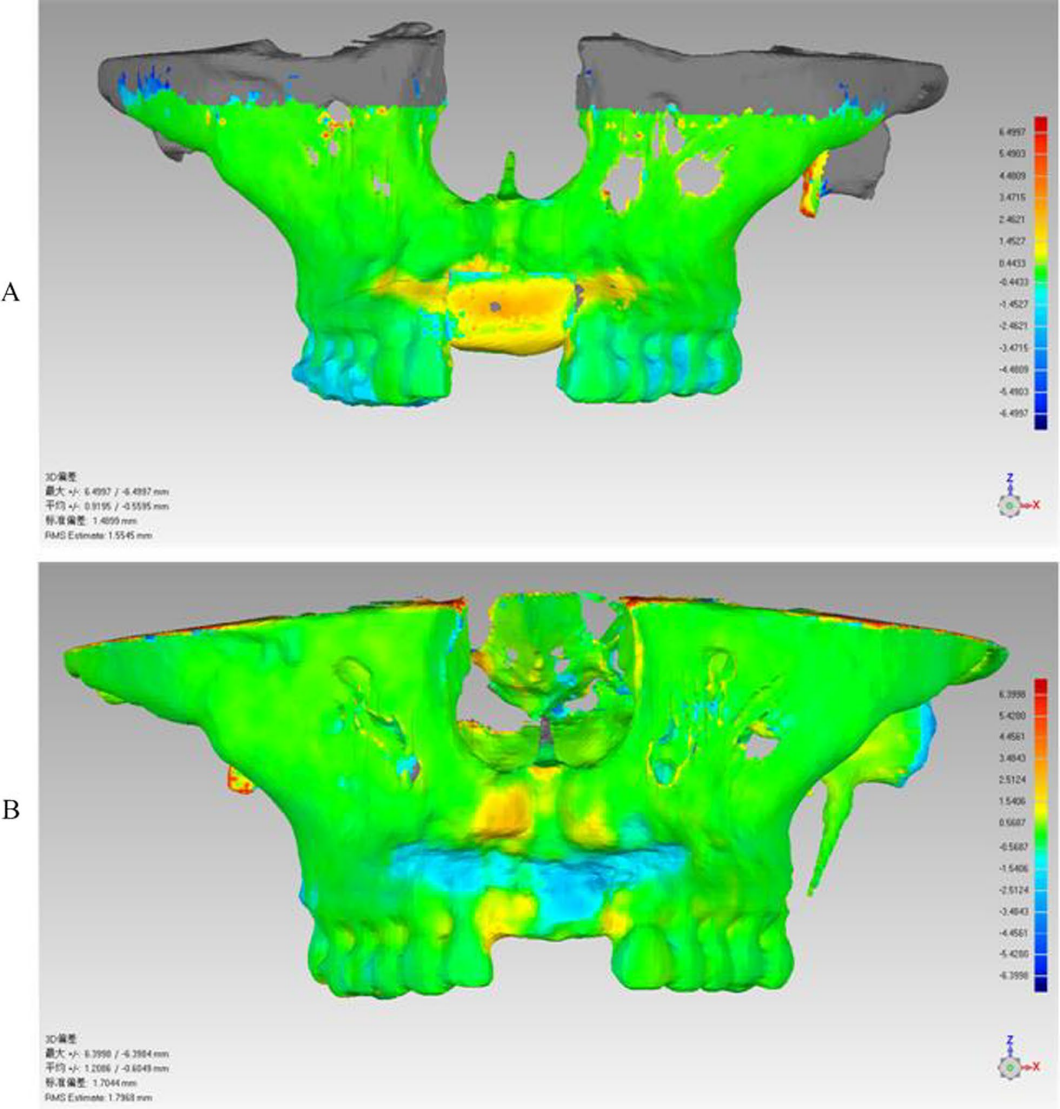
Color temperature chart of bone graft location: A comparison of CT results preoperatively and 2 weeks postoperatively, showing that the average error of bone position accuracy is 0.73 mm; B comparison of CT results between 2 weeks and 4 months postoperatively, showing bone resorption on the labial and alveolar ridge crest, especially on the labial side
